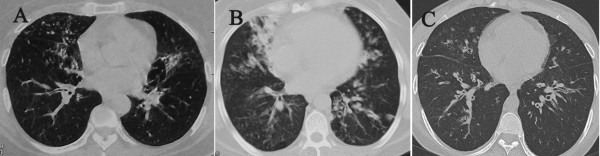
Chest CT of case 4 (A), 6 (B) and 7 (C), which demonstrates diffuse dilated and thickened bronchi accompanied with lung fibrosis.
